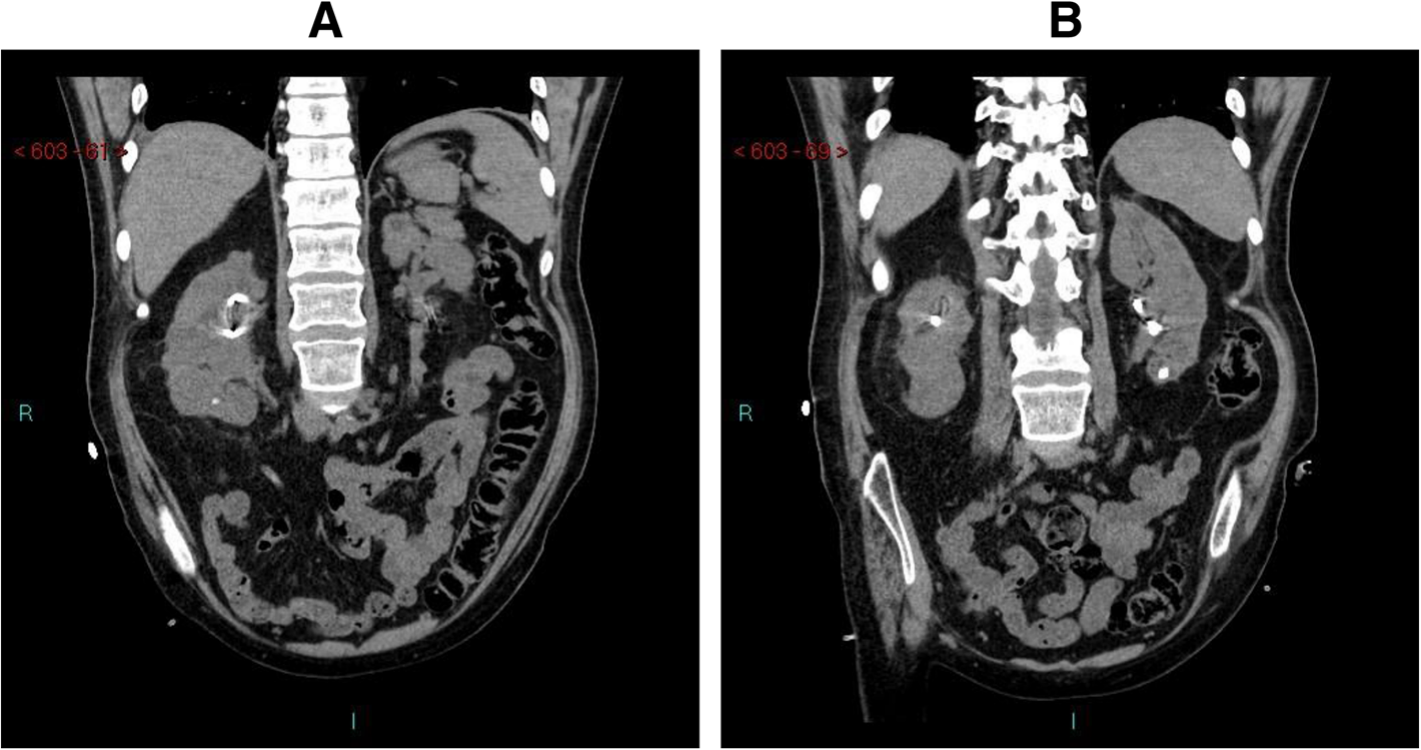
Case 2: Computed Tomography of kidneys, coronal view. (A) Right kidney: tiny calculus in lower pole; nephrostomy is in place. Outline of kidney is irregular due to cortical scarring. (B) Left kidney calculus in lower pole; nephrostomy is seen in place. Renal outline is irregular.
